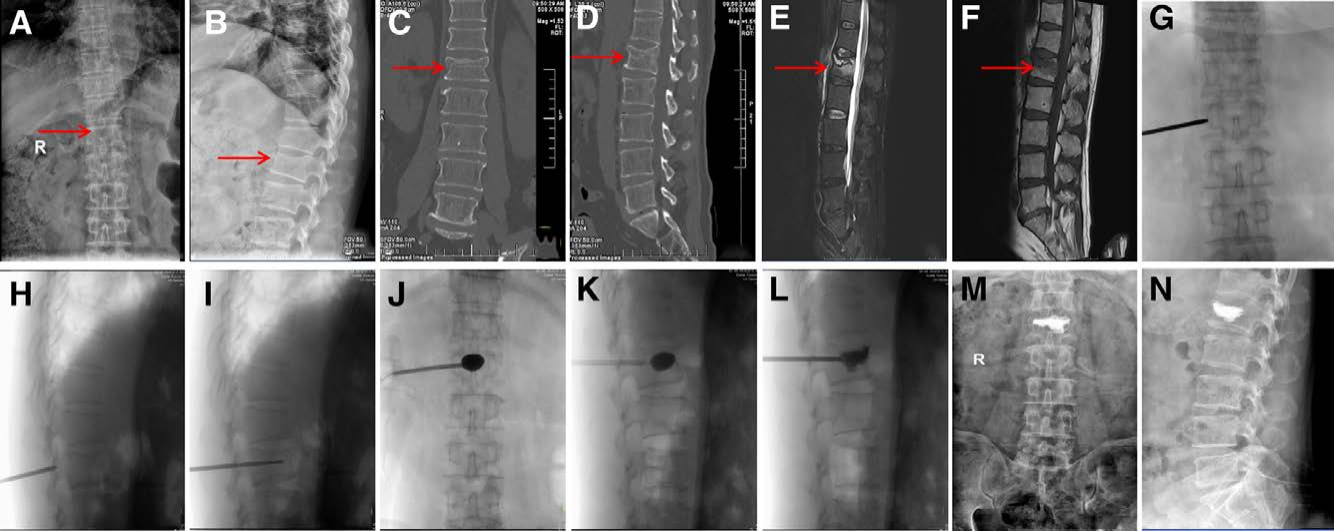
(A and B) X-ray examination shows L1 vertebral body compression. (C and D) CT scan reveals a fracture line below the superior endplate of the L1 vertebral body. (E) Sagittal MR T2-weighted image shows high signal changes (hemorrhage) at the fracture site and adjacent bone. (F) Sagittal MR T1-weighted image shows low signal changes (hemorrhage) at the fracture site and adjacent bone. (G–I) The unilateral pedicle outer wall is punctured first, followed by slow insertion of the channel into the vertebral body. A bone drill is then placed and drilled to the anterior one-third of the vertebral body. (J–L) After restoring vertebral height by balloon expansion, PMMA bone cement is slowly injected. (M and N) Postoperative follow-up X-rays (anteroposterior and lateral views) show restored vertebral height with no significant leakage of bone cement. CT = computed tomography, PMMA = polymethylmethacrylate (bone cement).
